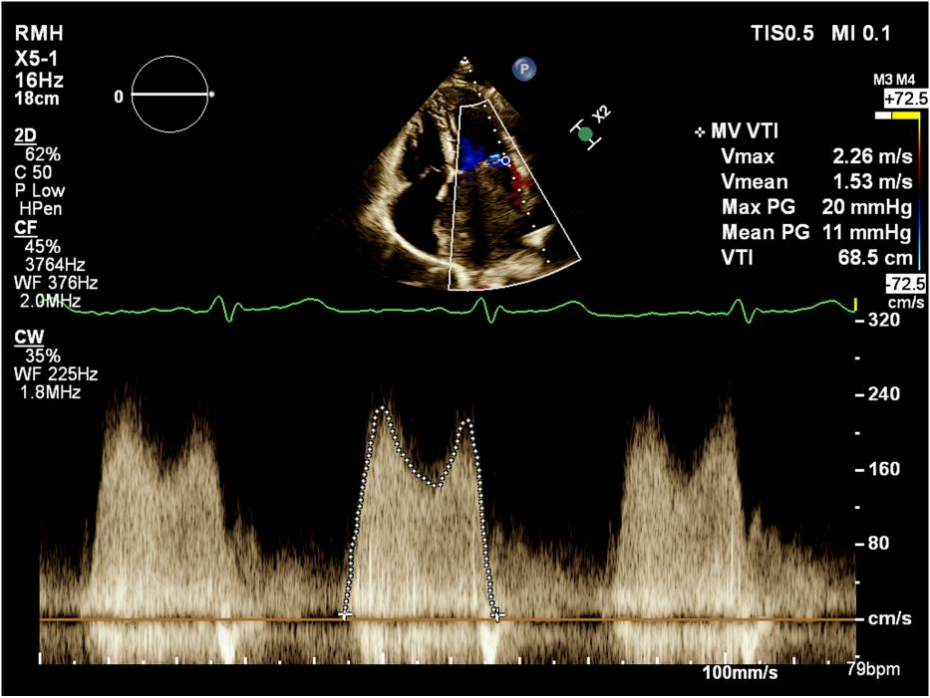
Transthoracic echocardiogram demonstrating massive left atrial mass with moderate-to-severe obstruction to flow through the mitral valve. Continuous wave Doppler across the mitral valve demonstrated a mean gradient of 11 mmHg and a peak gradient of 20 mmHg.
